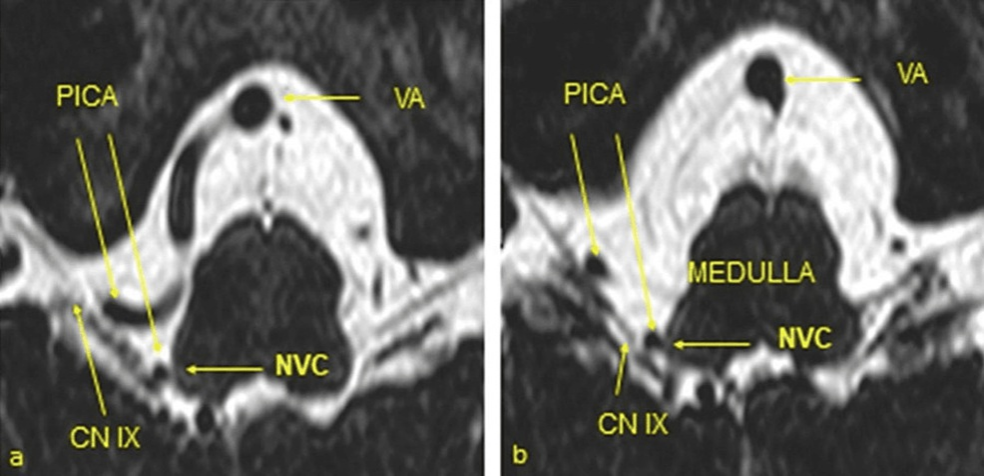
High-resolution, heavily T2-weighted MRI-CISS sequence with right-sided neurovascular compression (NVC) in right-sided glossopharyngeal neuralgia (a and b). Right-sided vascular loop of the posterior inferior cerebellar artery (PICA) originating from the vertebral artery (VA) with formation of an NVC at the root entry zone of the glossopharyngeal nerve (CN IX) at the ventrolateral medulla oblongata (CN: cranial nerve). Taken from Tanrikulu et al. with permission of the main author (published under Creative Commons license) [25].
